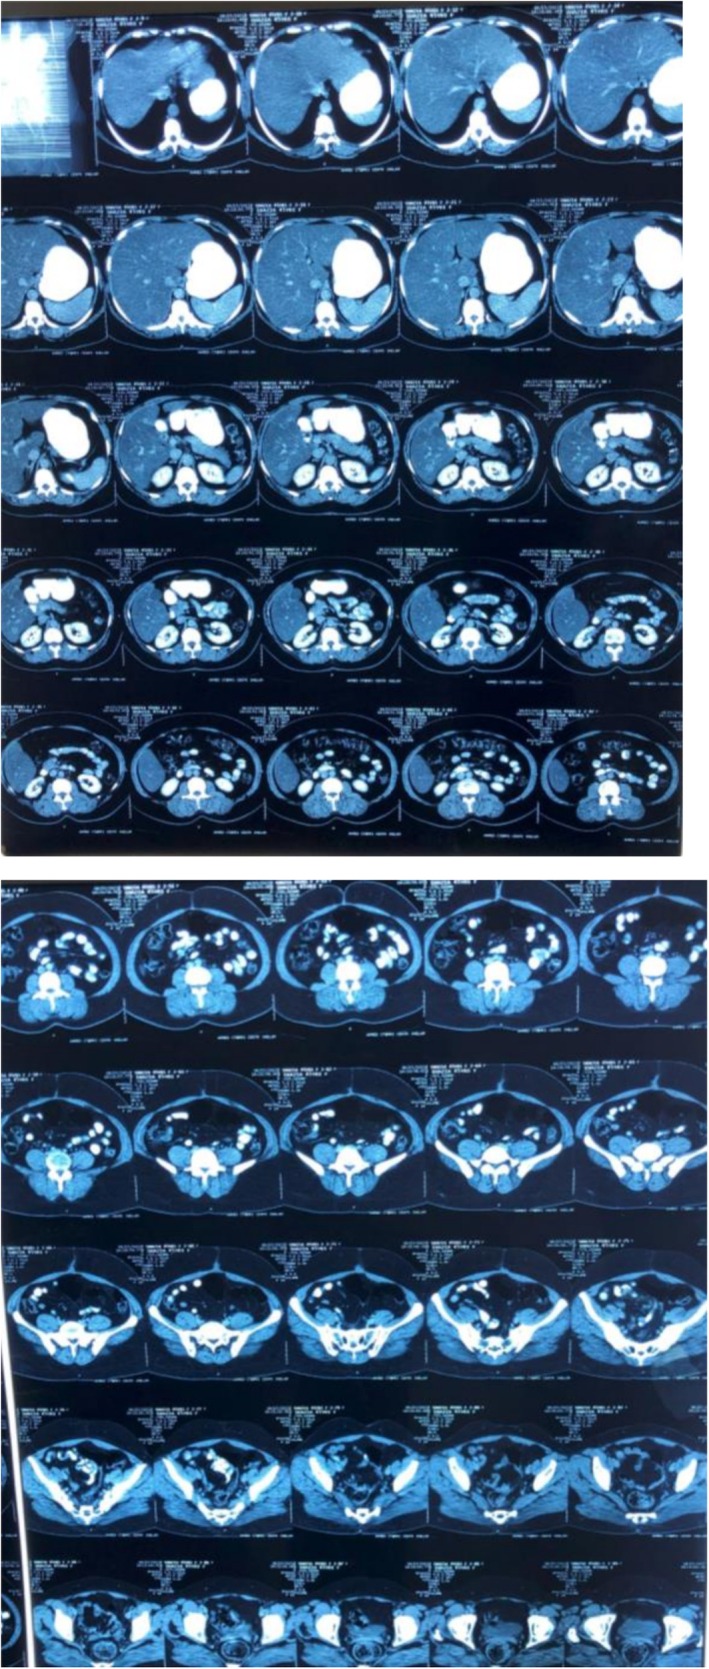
CT scan post four cycles of paclitaxel/carboplatin CT scan.
